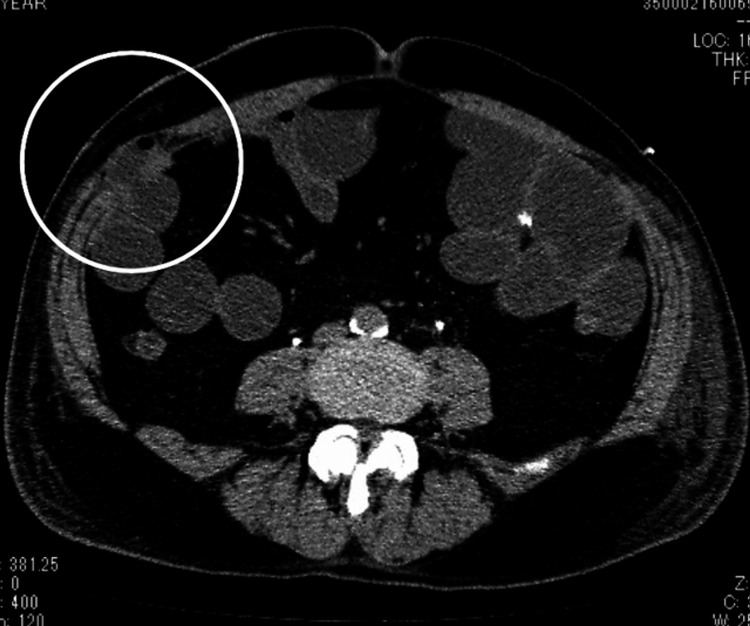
Abdominal computed tomography Computed tomography showed the small intestinal incarceration in the right abdomen.
